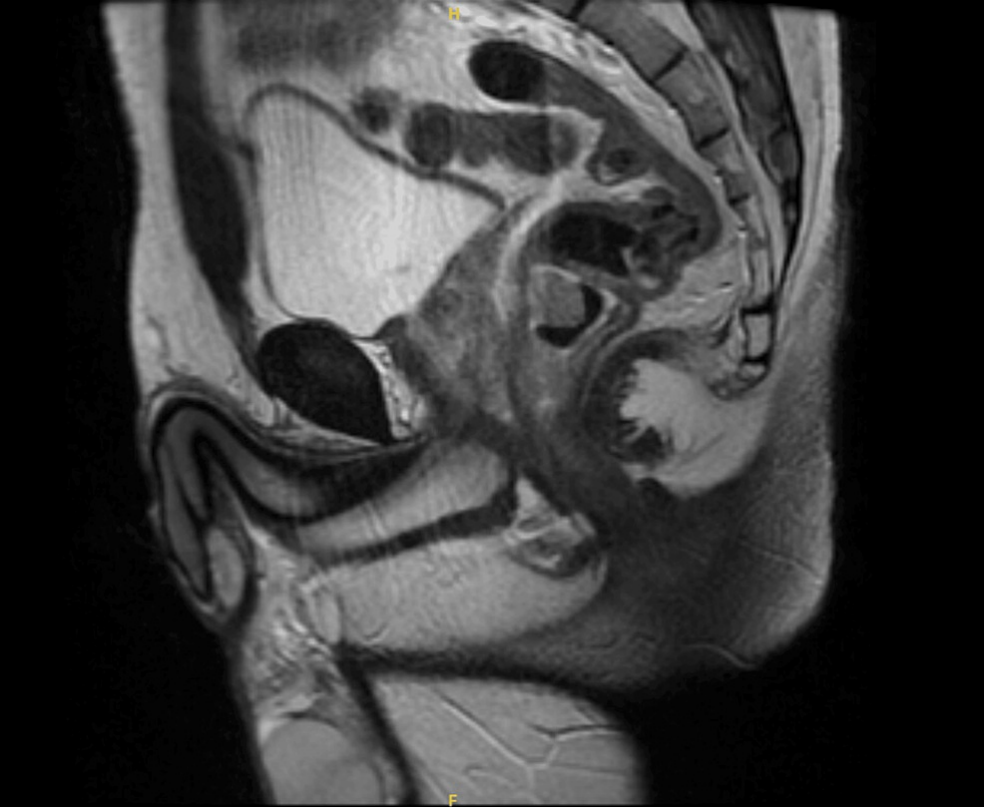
Normal MRI showing no involvement of perirectal fascia.
